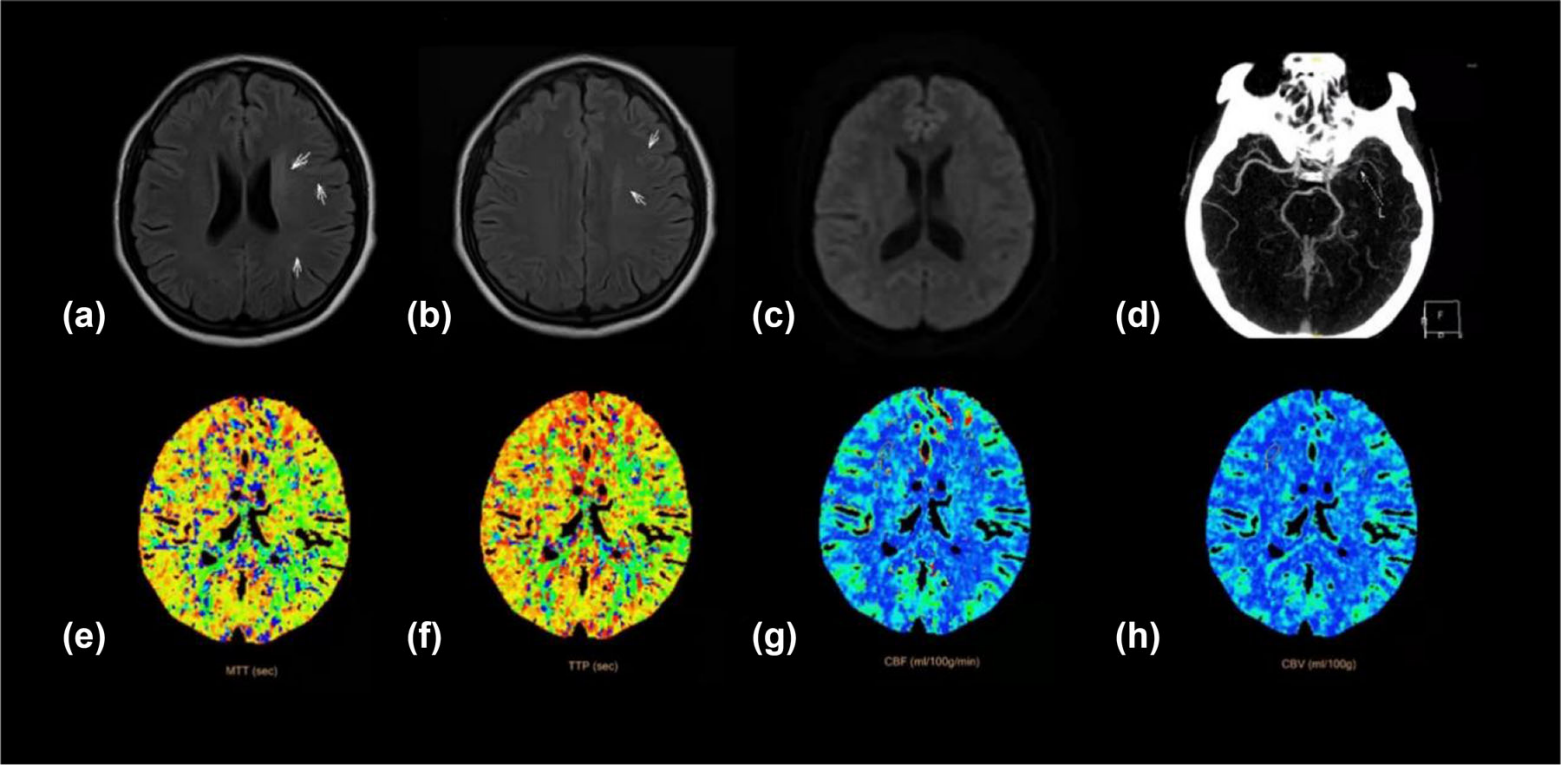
A patient with severe stenosis of the left middle cerebral artery (D), and stage II1 of cerebral perfusion (E, H). The left side has significantly more DWMHs and PWMHs than the right side (A, B); DWI did not show abnormalities (C).
